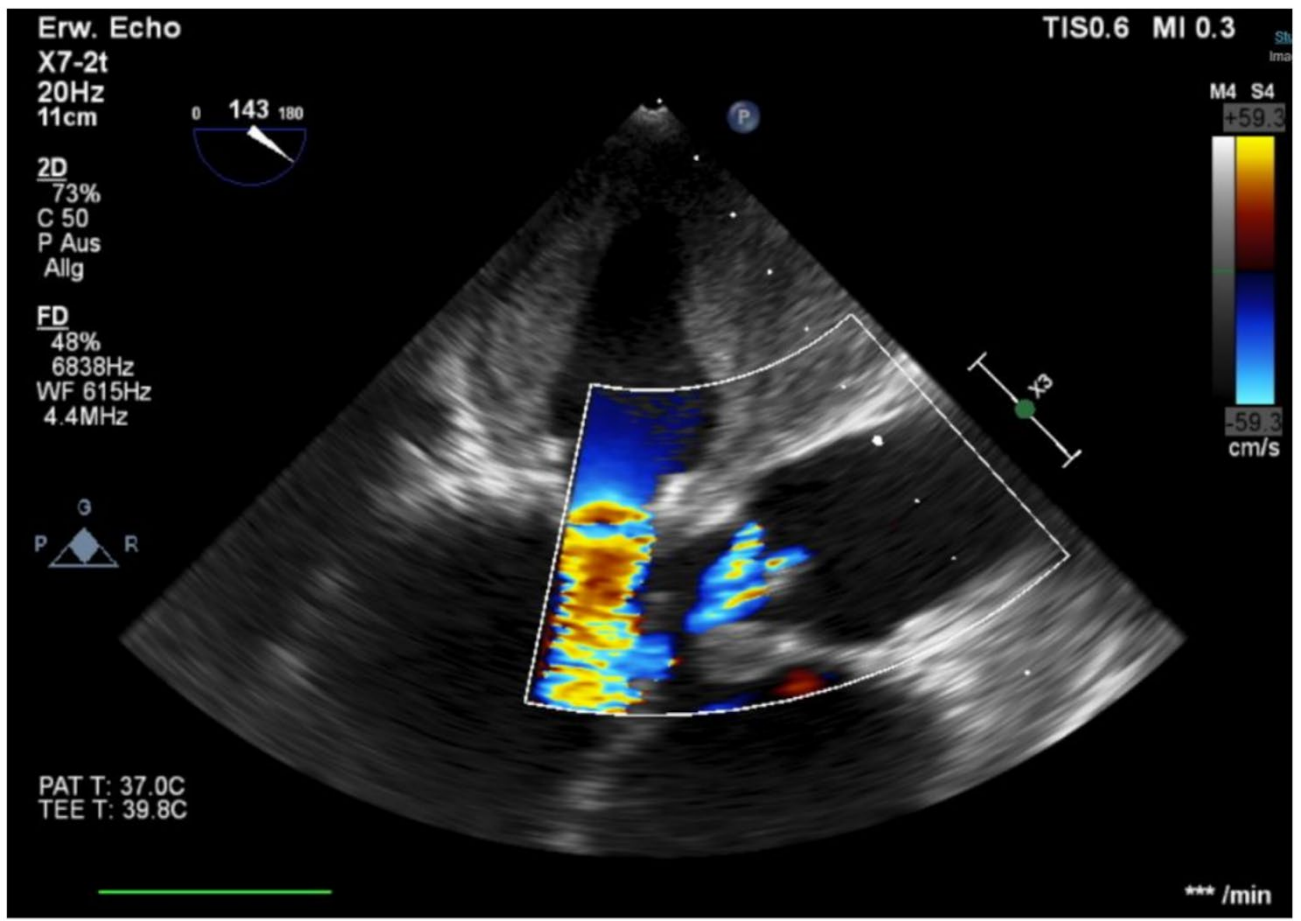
Doppler image of the mitral flow with candle flame phenomenon
